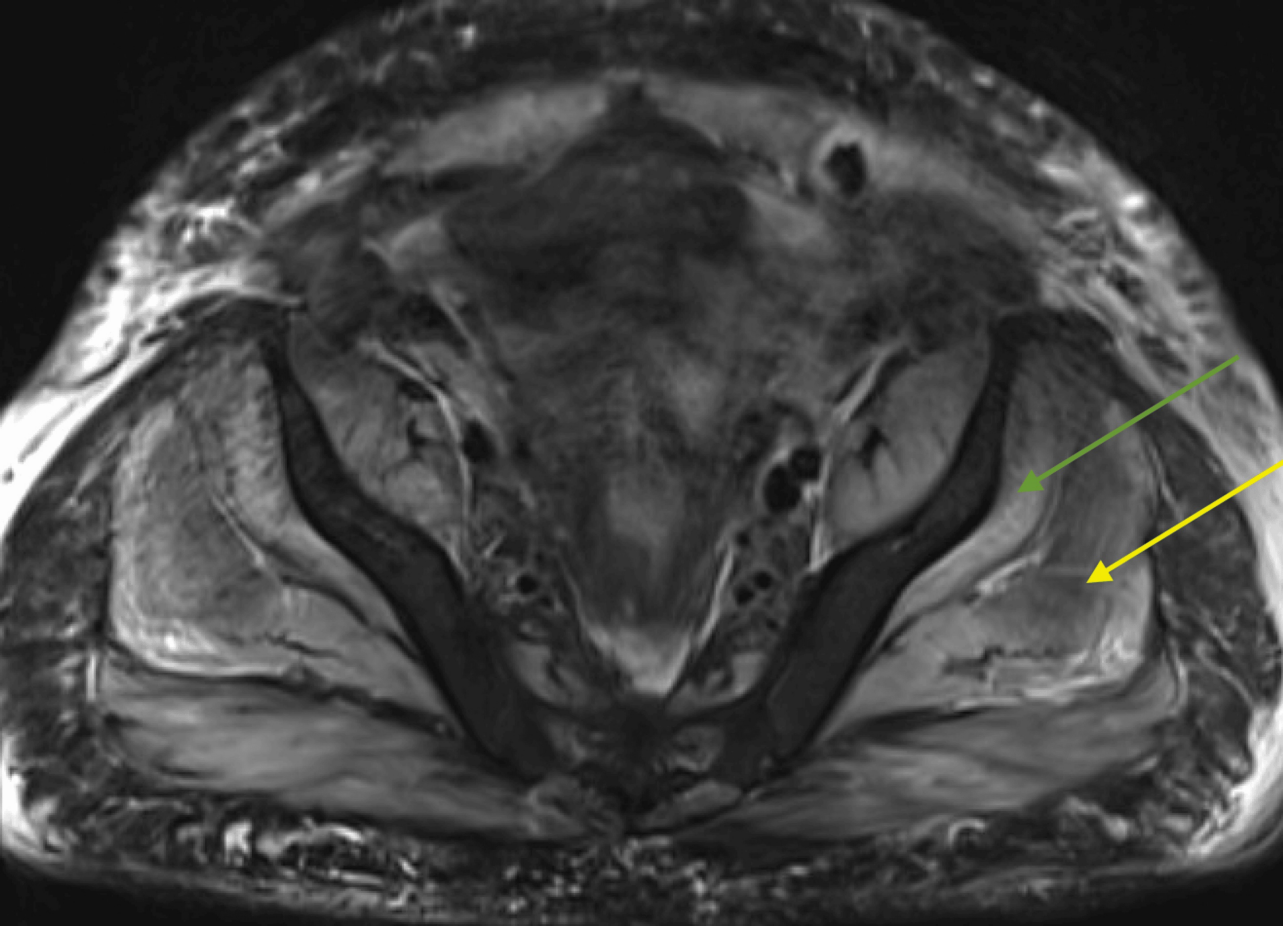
MRI of the left hip without contrast demonstrating muscle edema in the bilateral gluteus medius (yellow arrow) and gluteus minimus (green arrow).
